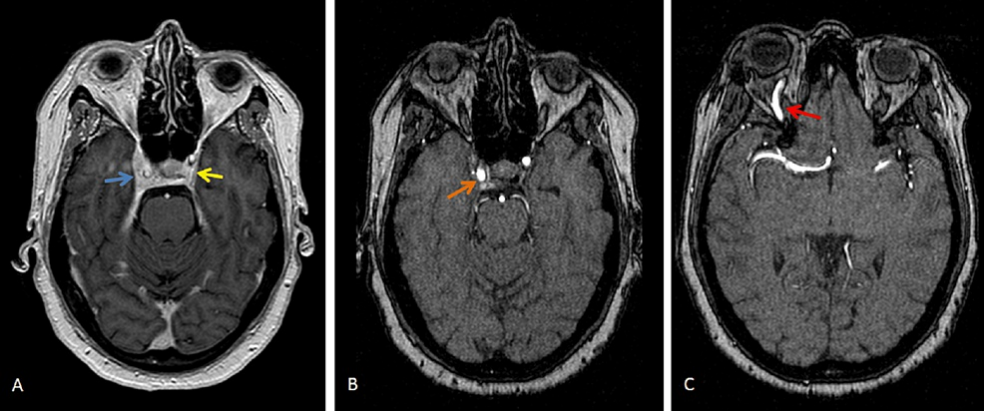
Brain angio MRI images of the patient. (A) Axial view showing the enlargement of the right cavernous sinus (blue arrow) compared to the opposite side (yellow arrow), (B) axial view showing the arterialization of the right cavernous sinus (orange arrow), and (C) axial view showing the arterialization of the right superior ophthalmic vein (red arrow).
